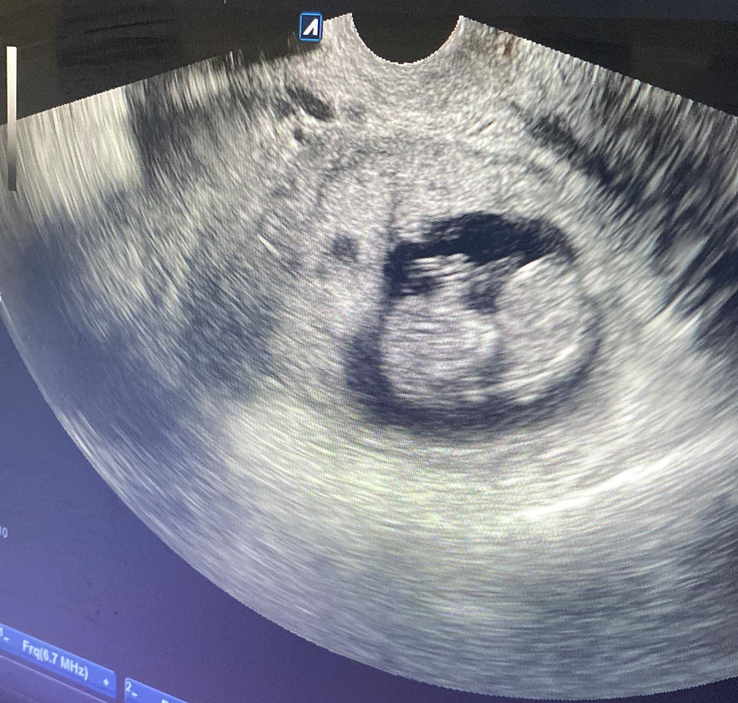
Trans-vaginal ultrasound showing uterine decidual reaction no gestational sac within the uterine cavity, and a gestational sac at the left fallopian tube pedunculated posterolateral and surrounded by myosalpinx.
